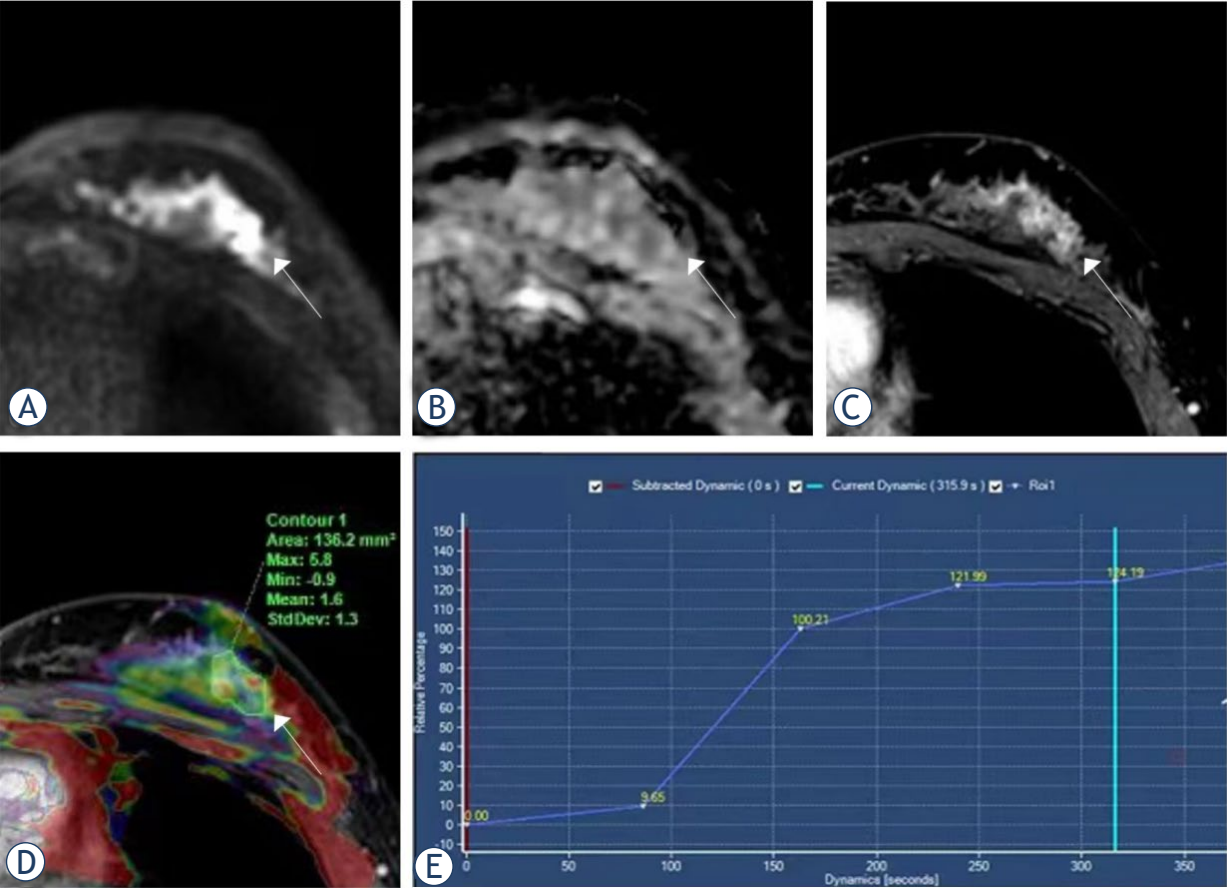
Magnetic resonance imaging (MRI) from a 26-year-old woman with intraductal papilloma. (A) Diffusion-weighted imaging (DWI); (B) apparent diffusion coefficient (ADC); (C) dynamic contrast-enhanced (DCE); (D) the amide proton transfer-weighted imaging (APTWI) pseudo-color image was merged with the DCE images and the APT value was 1.6%; (E) the type of time-intensity curves (TIC) was persistent.
